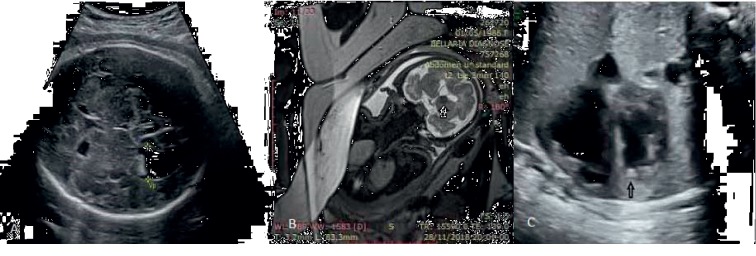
(a) Fetal ultrasound—bilateral hydrocephalus, mild on the right side and severe on the left side; (b) fetal MRI—lesion next to the foramen of Monro, typical for SEGA; (c) fetal ultrasound—echogenic intracardiac focus on the left ventricular apex.
